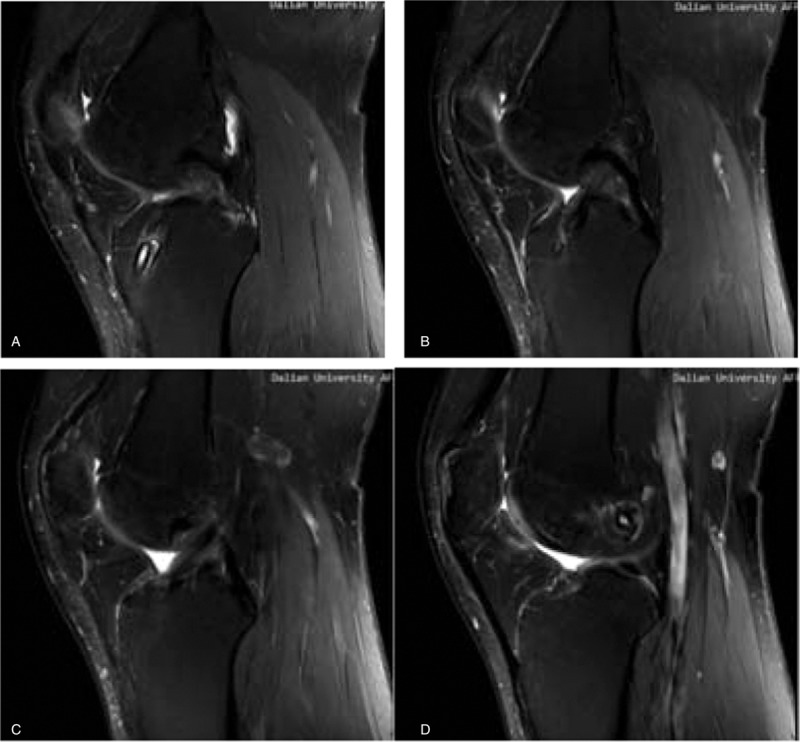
On oblique sagittal MRI. MRI = magnetic resonance imaging.
